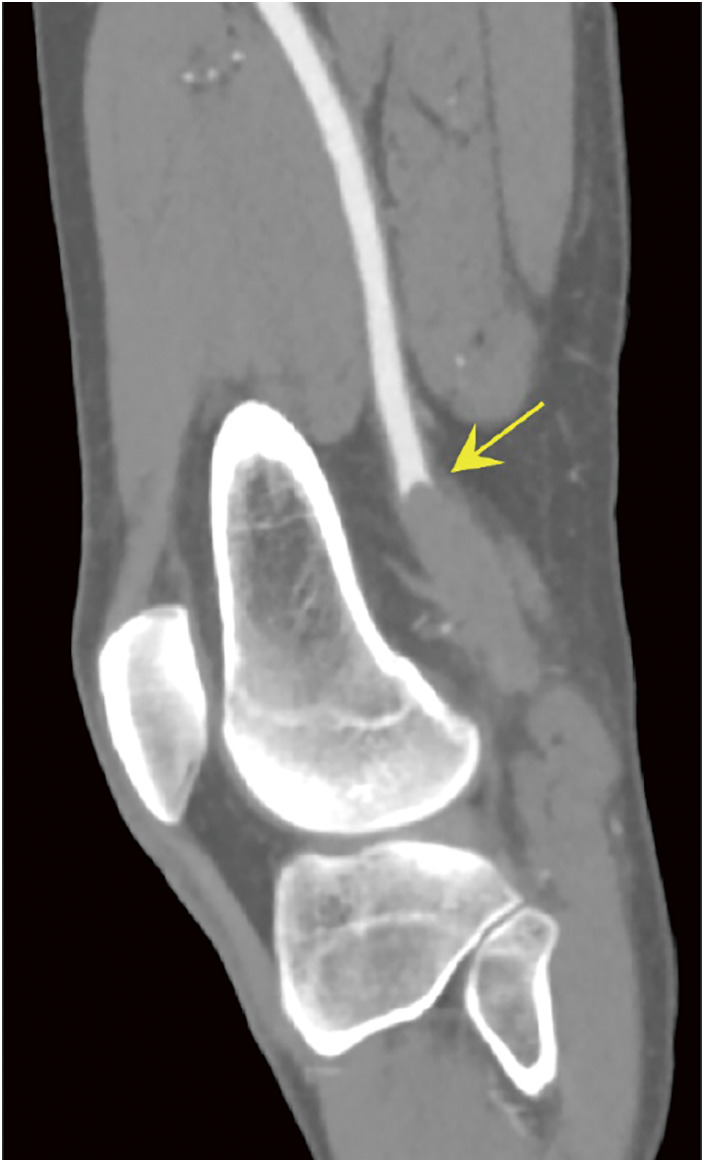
Fig. 1 Sagittal view of enhanced CT on initial presentation. The yellow arrow shows occlusion of popliteal artery. The top of thrombus is convex upward, which indicates acute occlusion. CT: computed tomography
